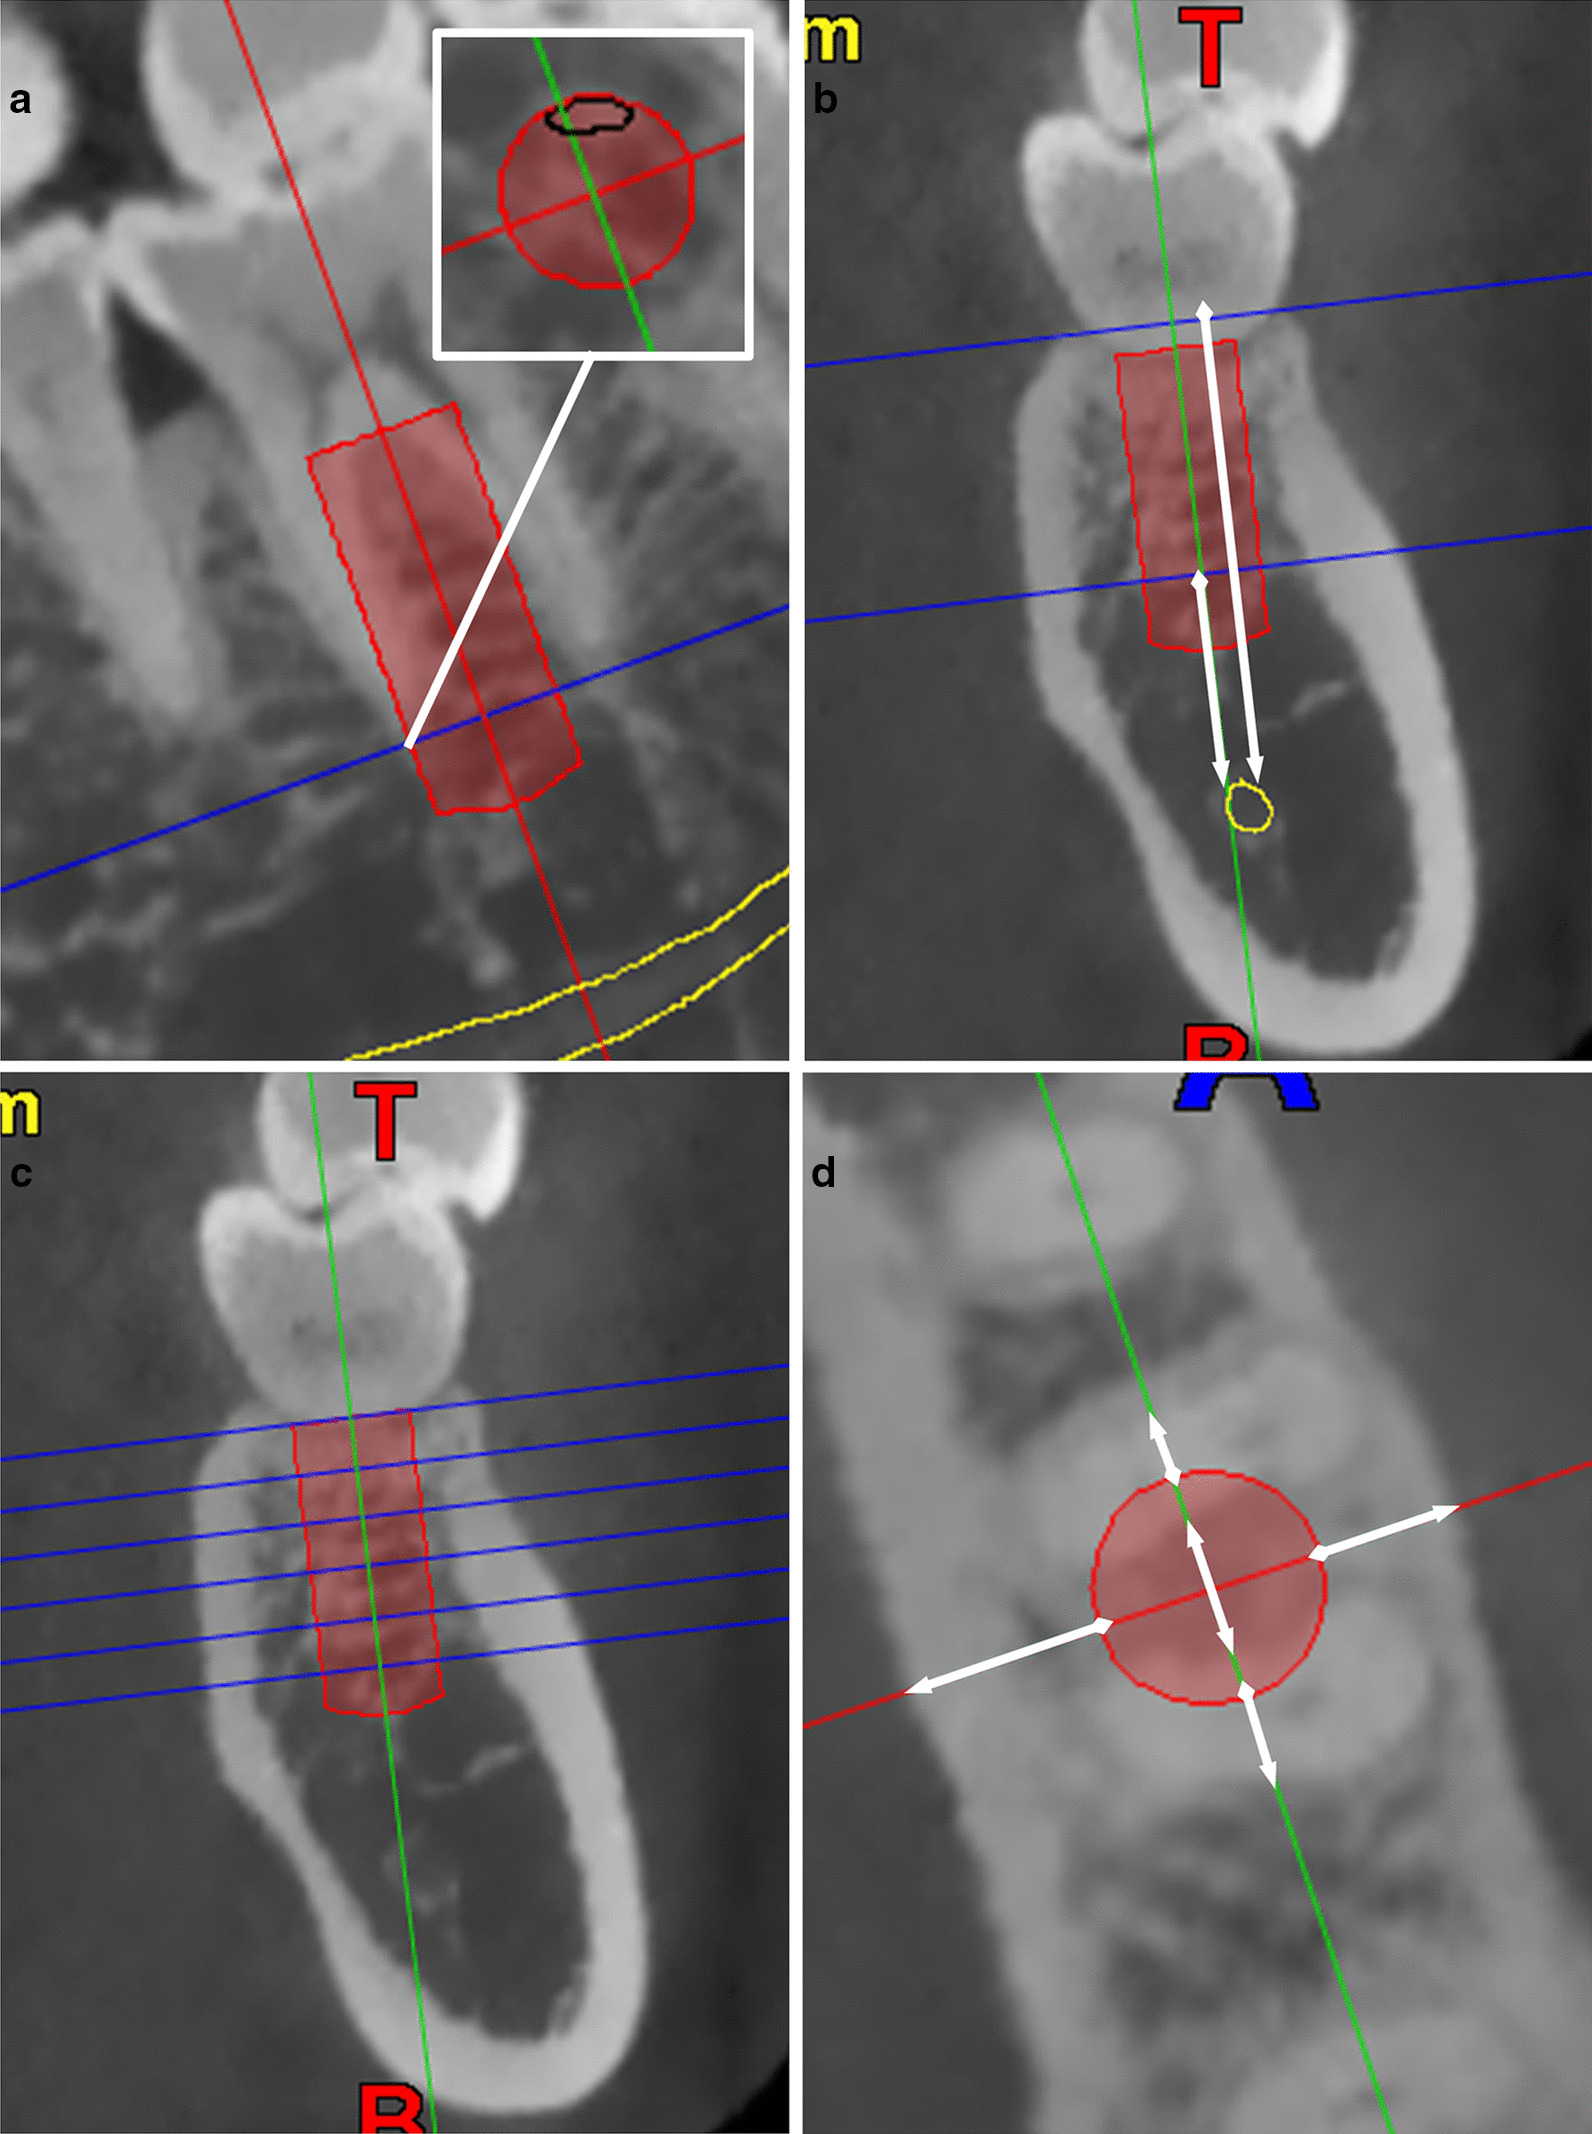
a Cross-sectional slice of the investigated molar, virtual implant was placed parallel to the long axis of the tooth (Straumann, bone level regular crossfit, 4.8 × 12 mm), and the level where the virtual implant was completely surrounded was identified. b The distance from the alveolar crest to the IAN, and the distance from the level where the virtual implant was completely surrounded by bone to IAN were measured. c CBCT sections 1, 3, 5, 7, 9, 11 mm apical to the alveolar crest were obtained. d Horizontal widths from the implant surface to the mesial and distal socket wall, the widths of inter-radicular septum, and the distances from implant surface to the buccal and lingual plate in each section were measured
